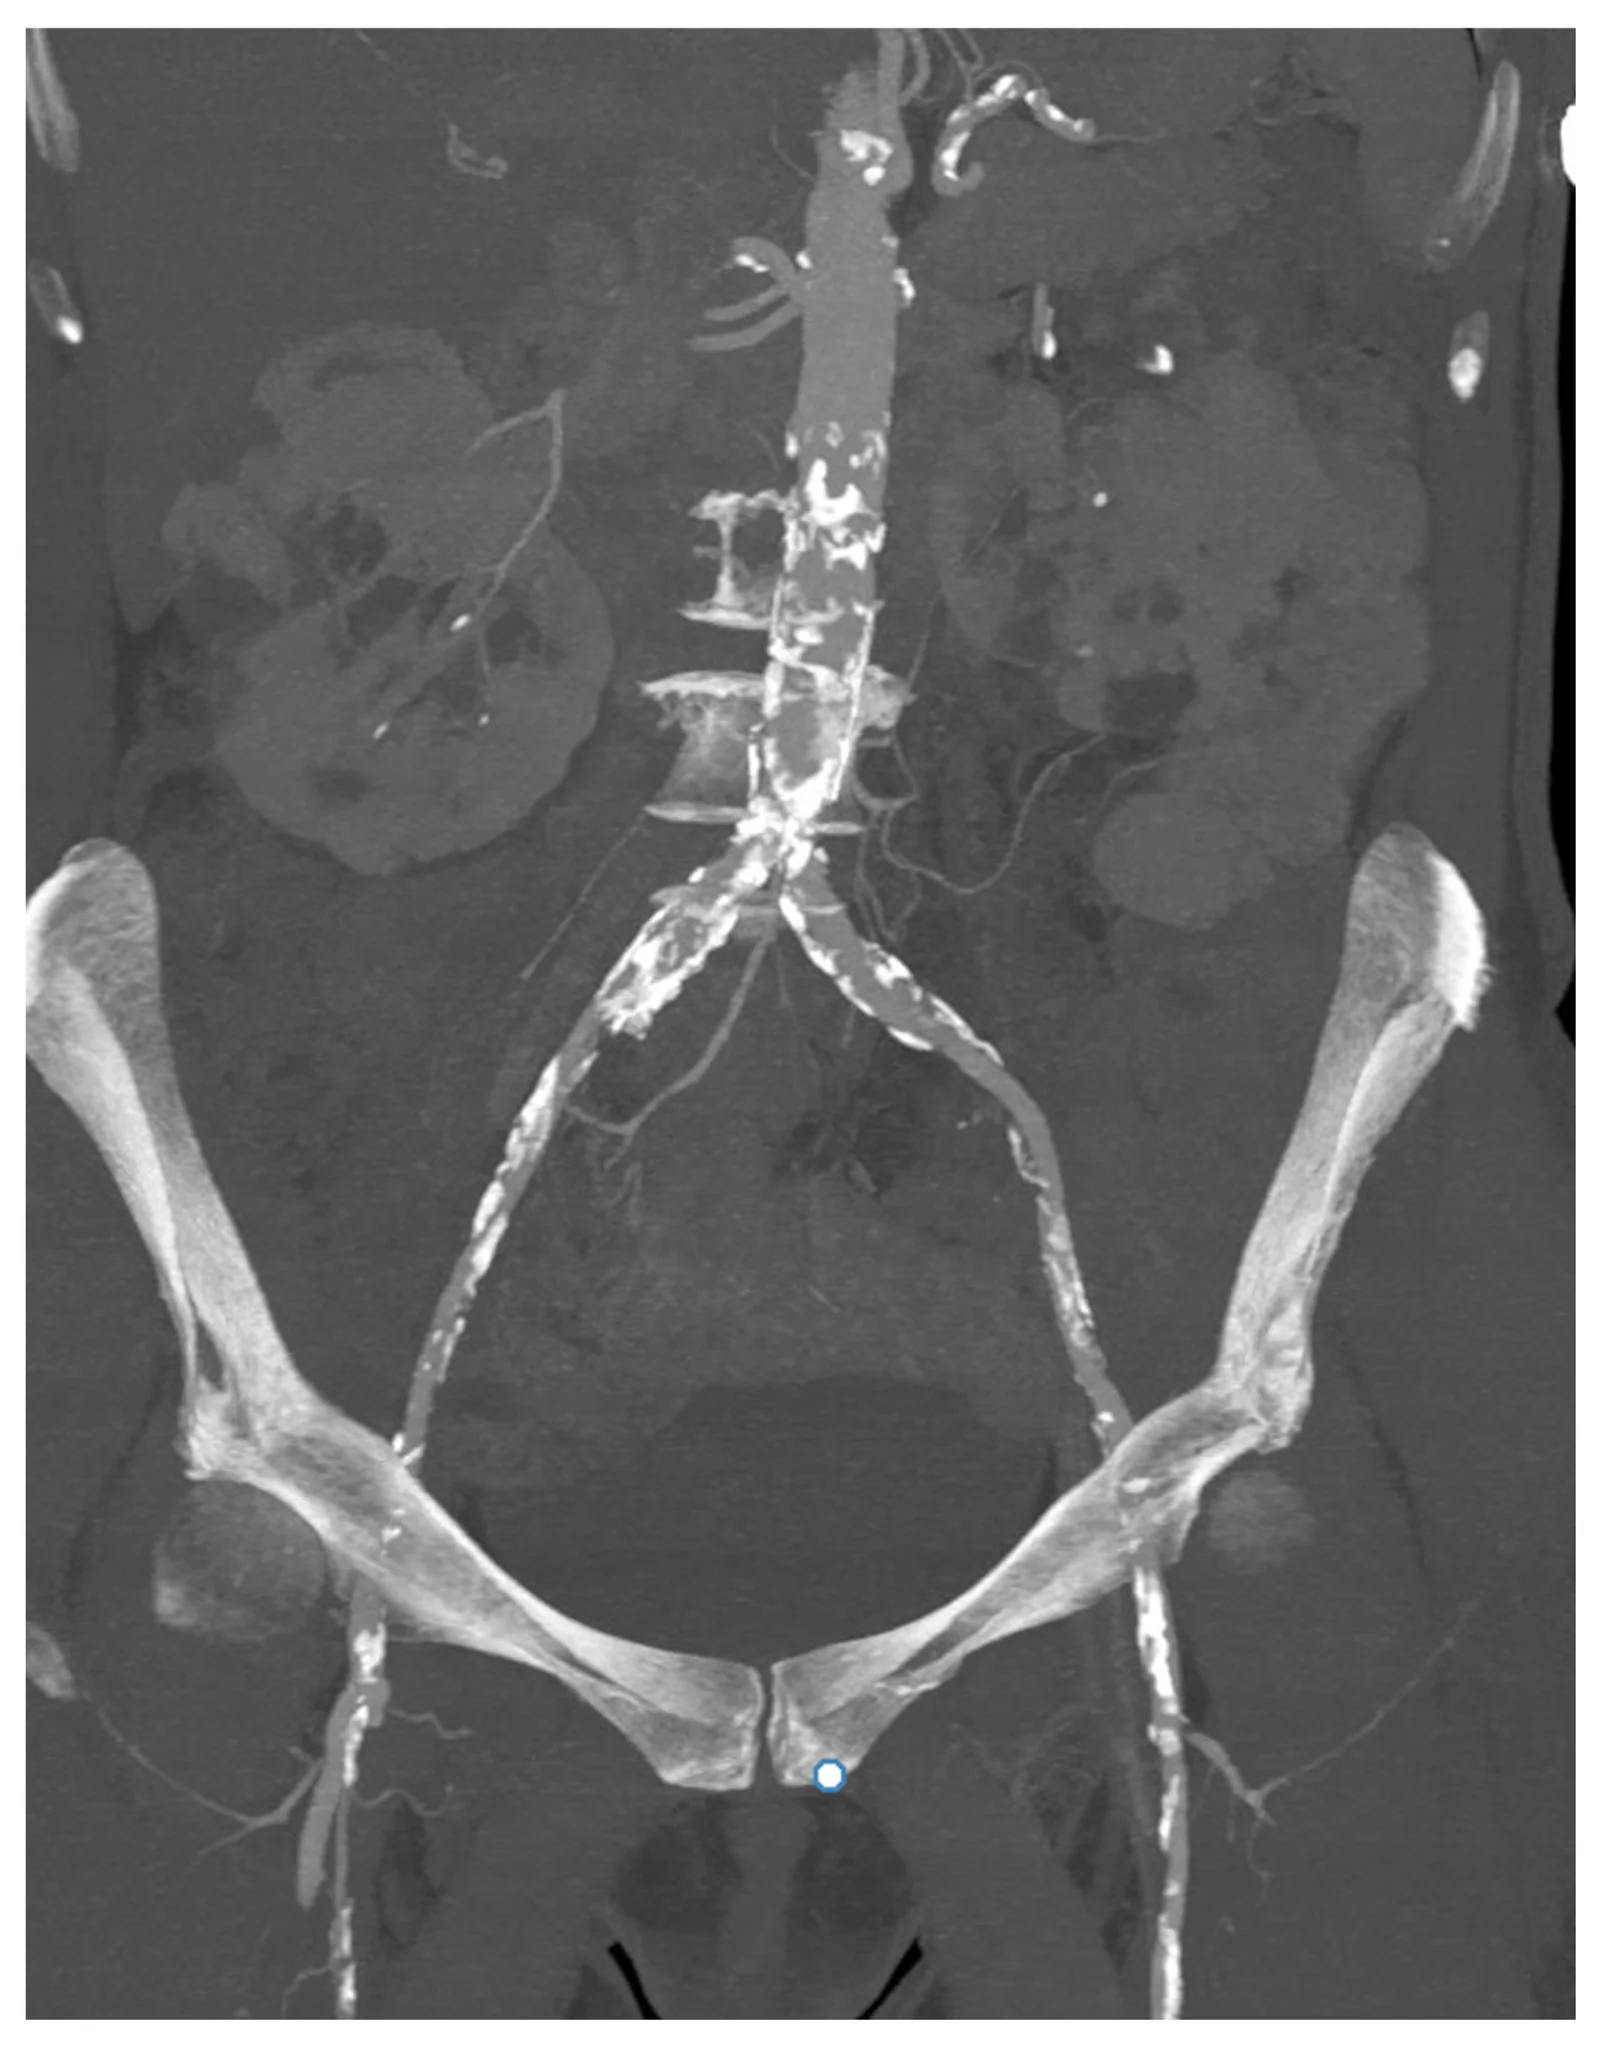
Computed tomography angiography of iliofemoral arteries.
